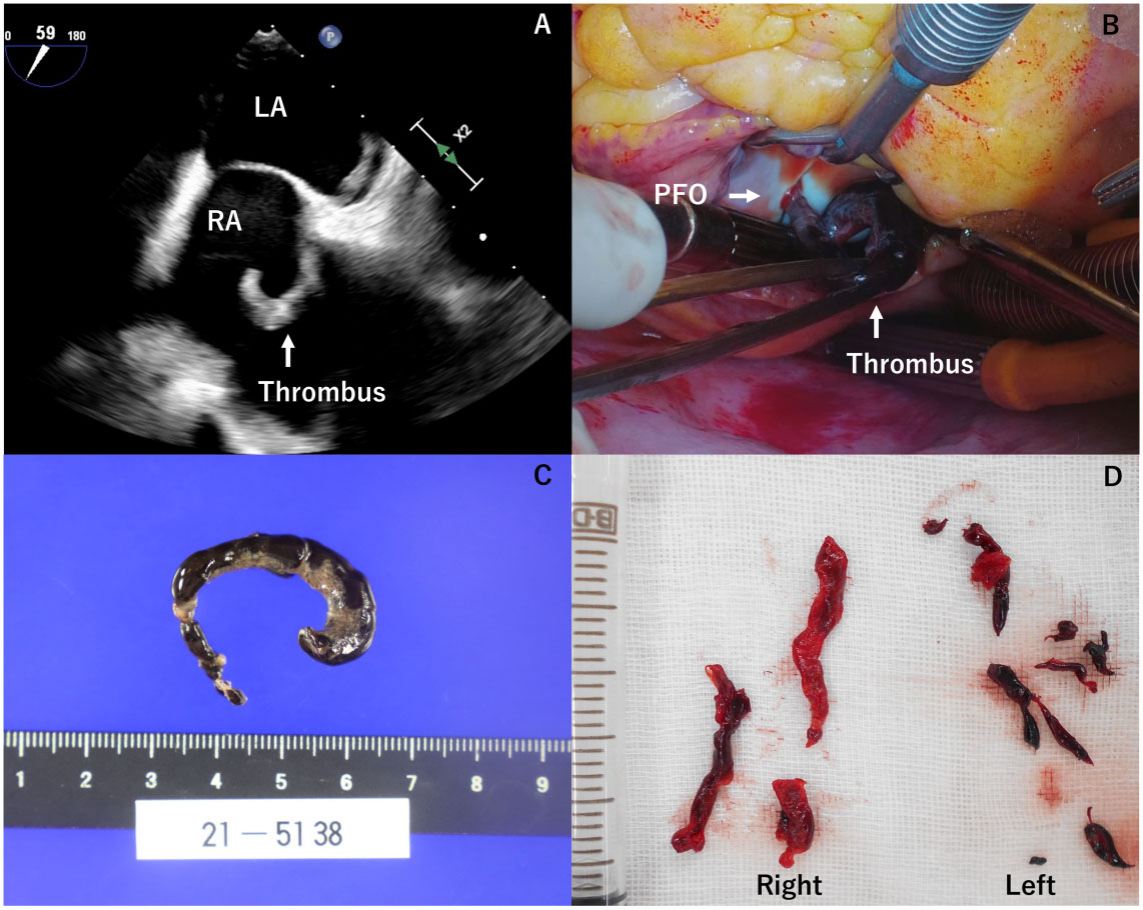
(A) Transoesophageal echocardiography, worm-like thrombus (arrows) straddling the PFO in both atria. (B) Clot retrieved at embolectomy. A worm-like thrombus was lodged into the PFO. (C) Pathologically, the thrombus has no malignant findings. LA: left atrium; PFO: patent foramen ovale; RA: right atrium. (D) Clot retrieved at thrombectomy by aspiration using a catheter. White and red thrombi were removed from the right PA. Many red thrombi were removed from the left PA. (A color version of this figure appears in the online version of this article.)
